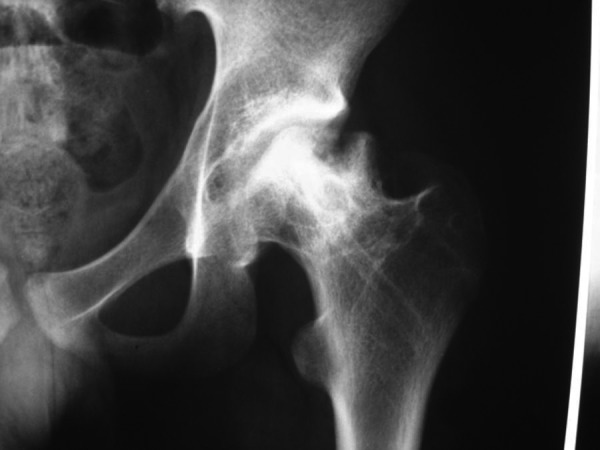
Radiography of the Hip in the Same Patient After Three Years and Removal of Screws
